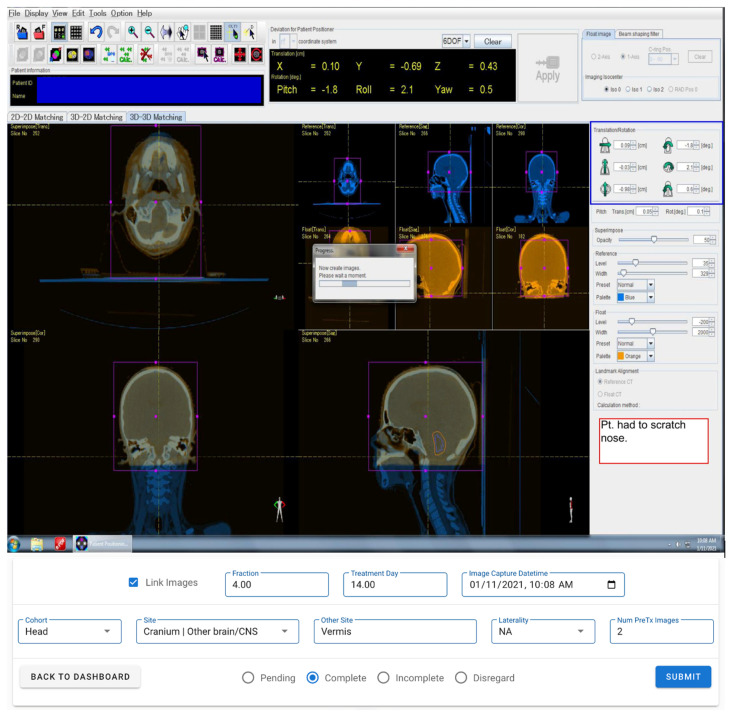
An example screen capture of Patient Positioning and Image Analysis (PIAS) software (v5.3.0), stored for each patient CBCT scan in our custom data management and manual review application. Setup corrections or residual errors (blue boxes) in CBCT coordinates and special conditions (red box) entered by therapists are captured using optical character recognition. Fractional and cohort information are queried from record and verify system queued for manual review in the application (form inputs at bottom).
